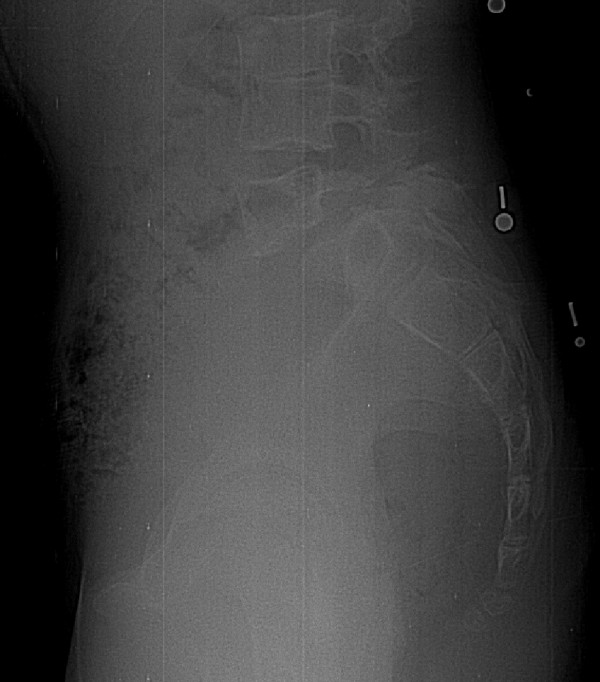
A case associated with disagreement between observers regarding the slip grade. Only the lumbosacral region from the original radiographs is provided.
